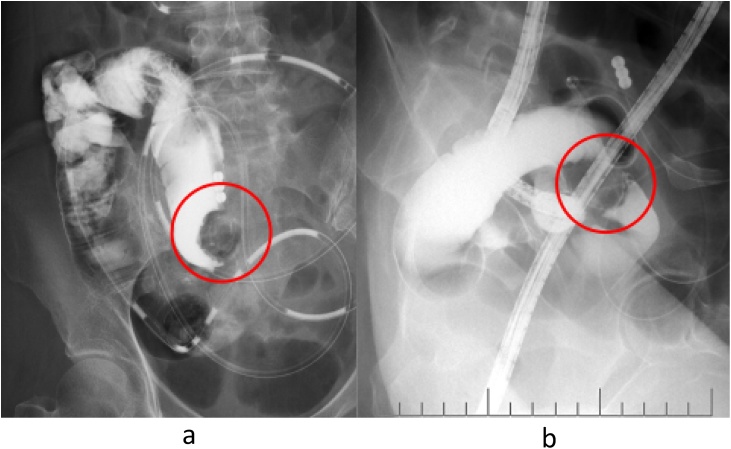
Radiographic findings. After insertion of an ileus tube and contrast infusion, a crab-like stenosis was found at the terminal ileum at the same site as the computed tomography image (a). Transanal double balloon endoscopy showed narrowing and contrast findings of 25 mm in size, 8 cm from the terminal ileum (b).
